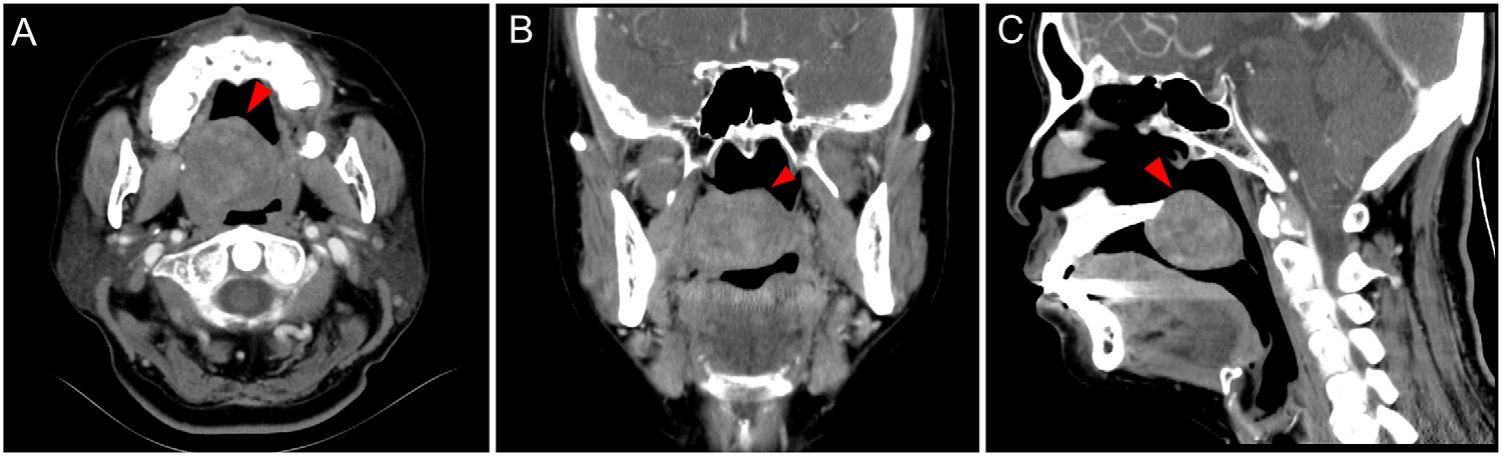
Enhanced CT images of the neck in a patient with palatal myoepithelioma.
